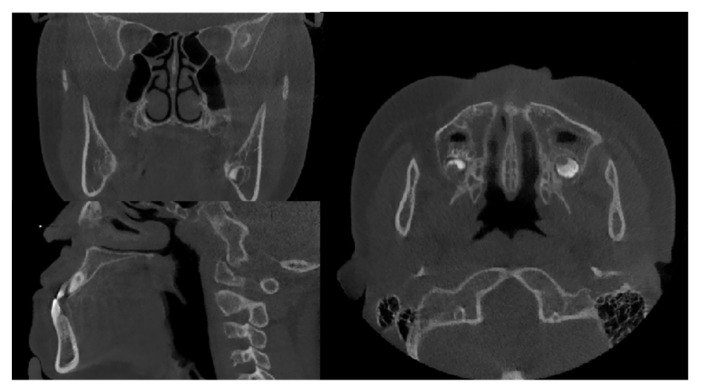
The image example of sagittal, coronal, and axial views of one CBCT file.
